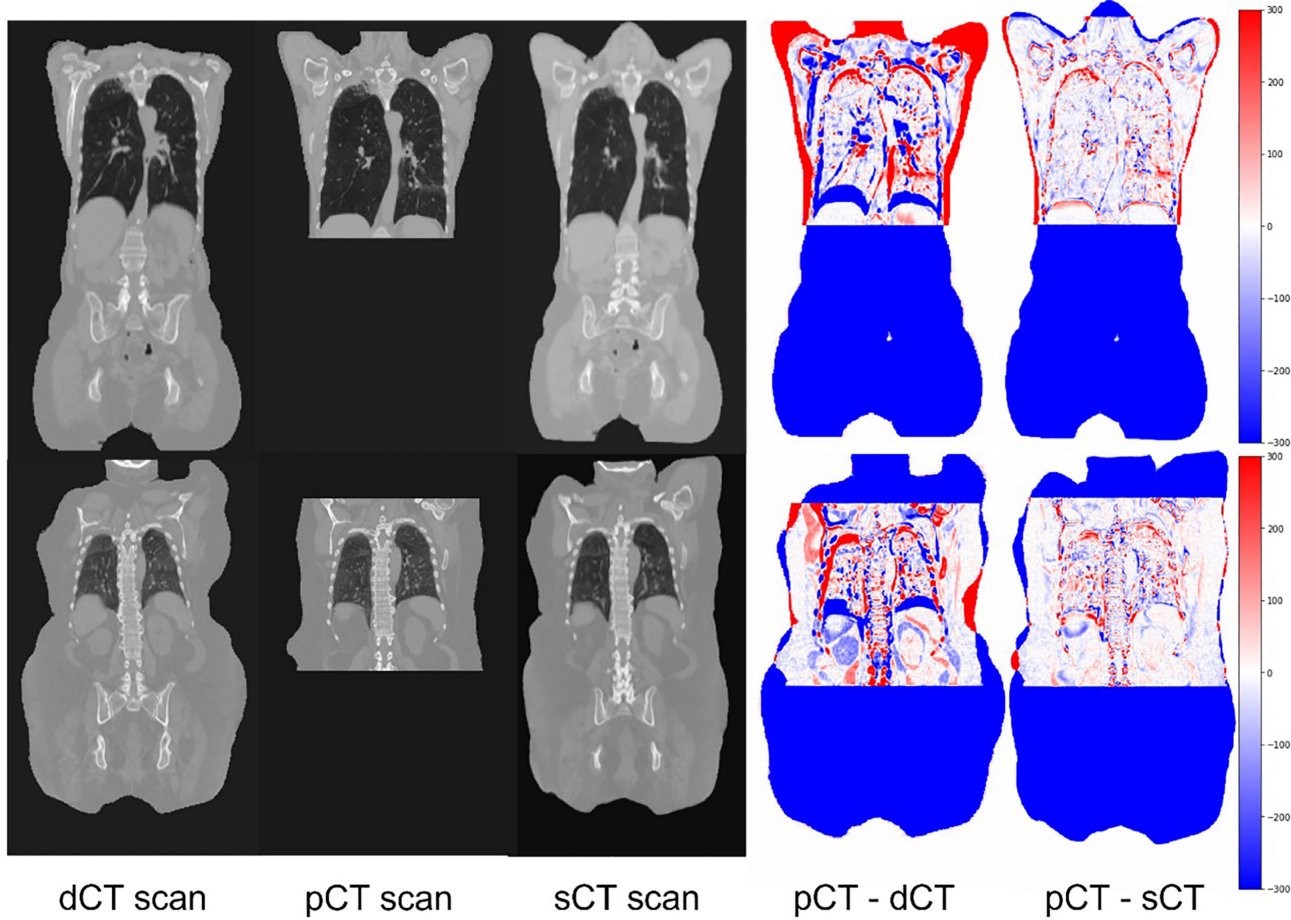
Example coronal slides extracted from two sample test cases. The input image, dCT (PET/CT scan), target scan (pCT), warped scan using the deepPERFECT generated DVFs (sCT), difference image pCT–dCT (target–input scan) (fourth column), and difference image pCT–sCT (target–output scan). The output scans high conformality with the target image for the overlapping area. Most prominently, the diaphragm level and lung bronchus, which are mainly affected by the ABC technique, are well aligned on sCT and target pCT scans.
